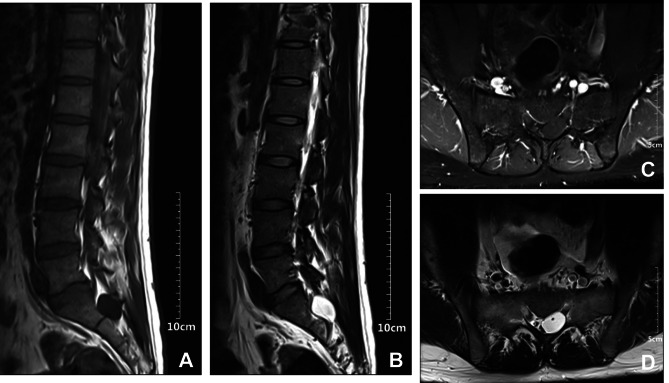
Lumbar magnetic resonance imaging revealed a Tarlov cyst at the left of S1 level in the sacral canal. (A) MRI T1 sugittal. (B) MRI T2 sugittal. (C) MRI T1 axial. (D) MRI T2 axial
